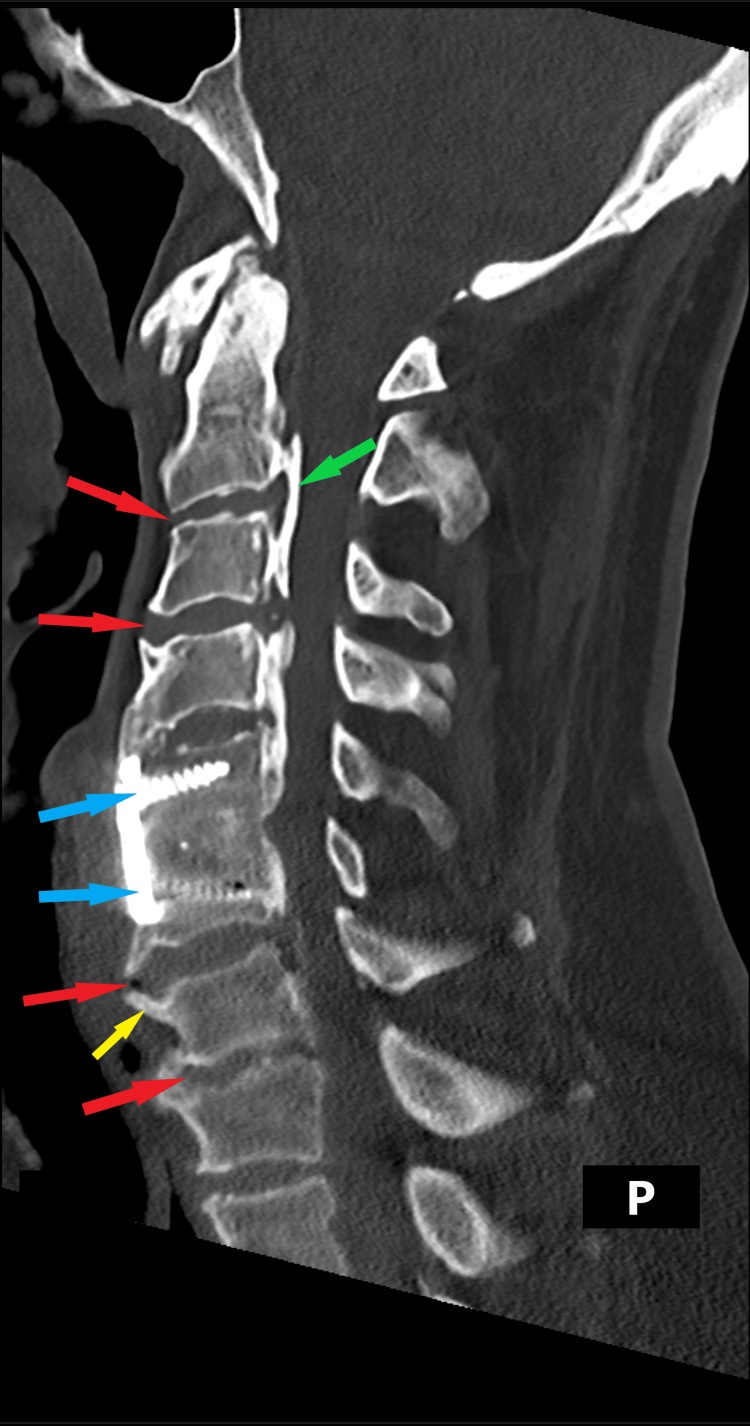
Sagittal computed tomography (CT) of the cervical spine without contrast shows postsurgical change from a prior anterior cervical discectomy and fusion (ACDF) procedure at the C5-C6 level with vertebral screws (blue arrows). It demonstrates multilevel disc space narrowing at the C2-C3, C3-C4, C6-C7, and C7-T1 levels (red arrows). Ossification of the posterior longitudinal ligament is present (green arrow). Degenerative spurring is noted (yellow arrow).
